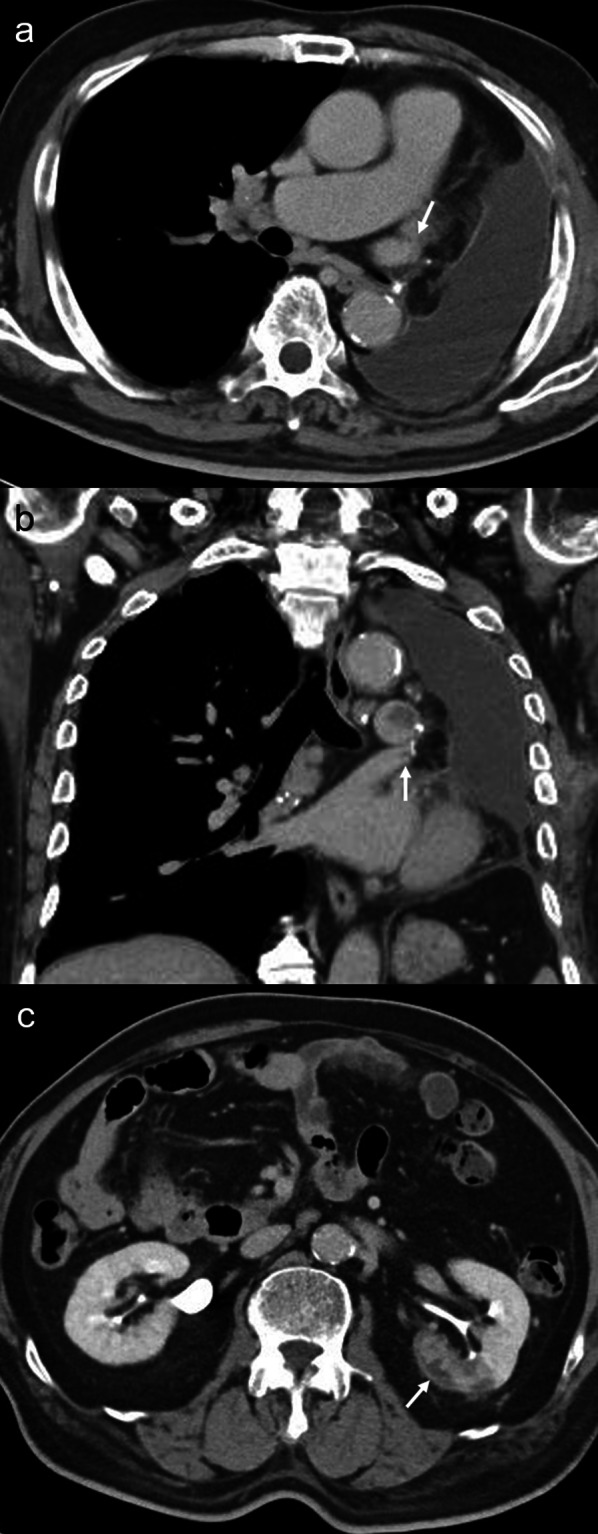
A contrast-enhanced computed tomography on postoperative day 19 reveals a reduction of the thrombus in the left upper pulmonary vein stump: a coronal view, b horizontal view, and c recovery of the partial defect of the left kidney
